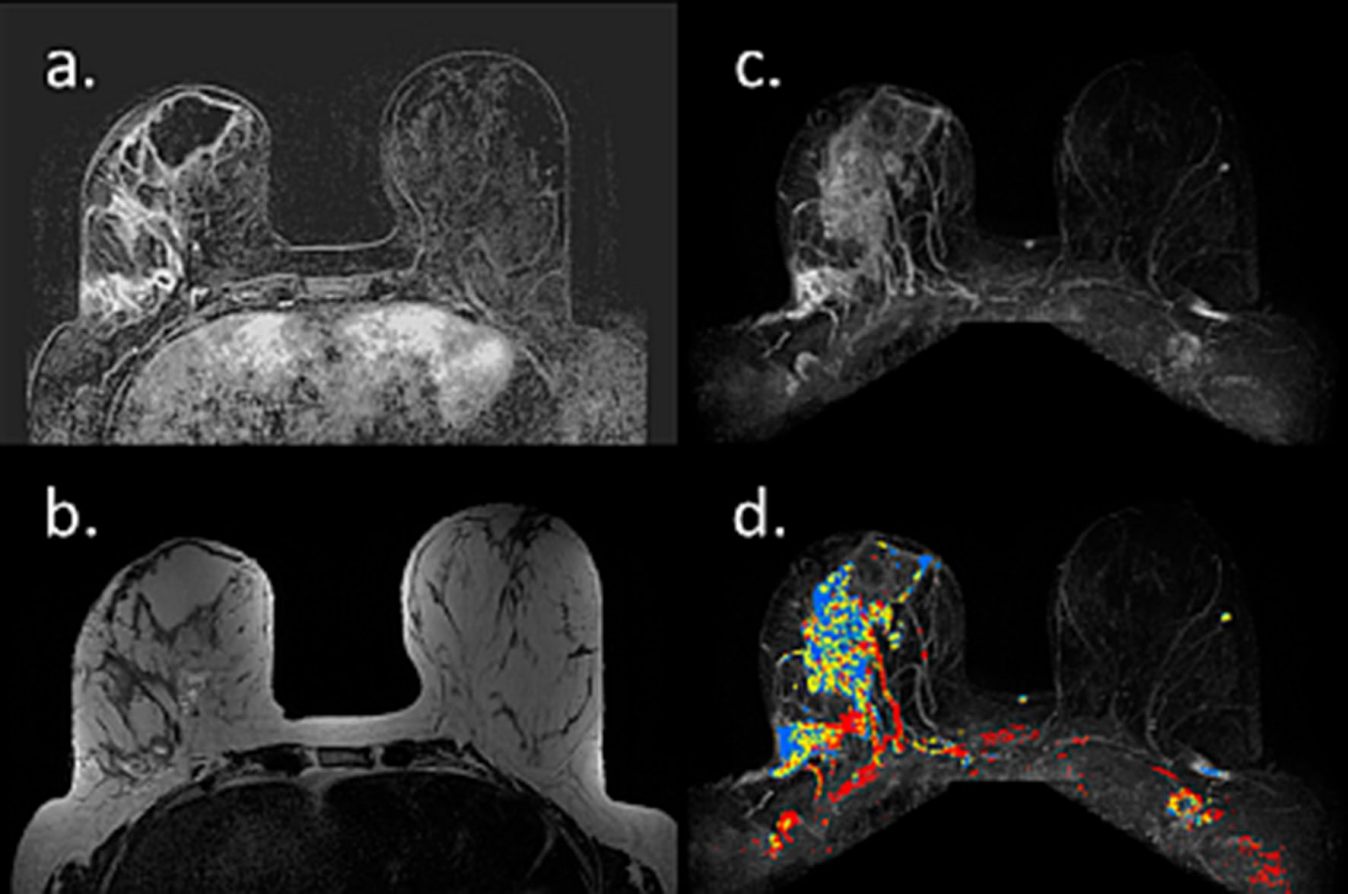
Abscess. MRI of 31-year-old patient with a history of recurrent breast mastitis and abscesses during several separate lactation periods is presented, this time in aspiration-confirmed breast abscess post weaning. Subtracted DCE (a) MIP (c) and CAD (d) reveal large rim enhancing regions with high T2 signal (b), compatible with an abscess
